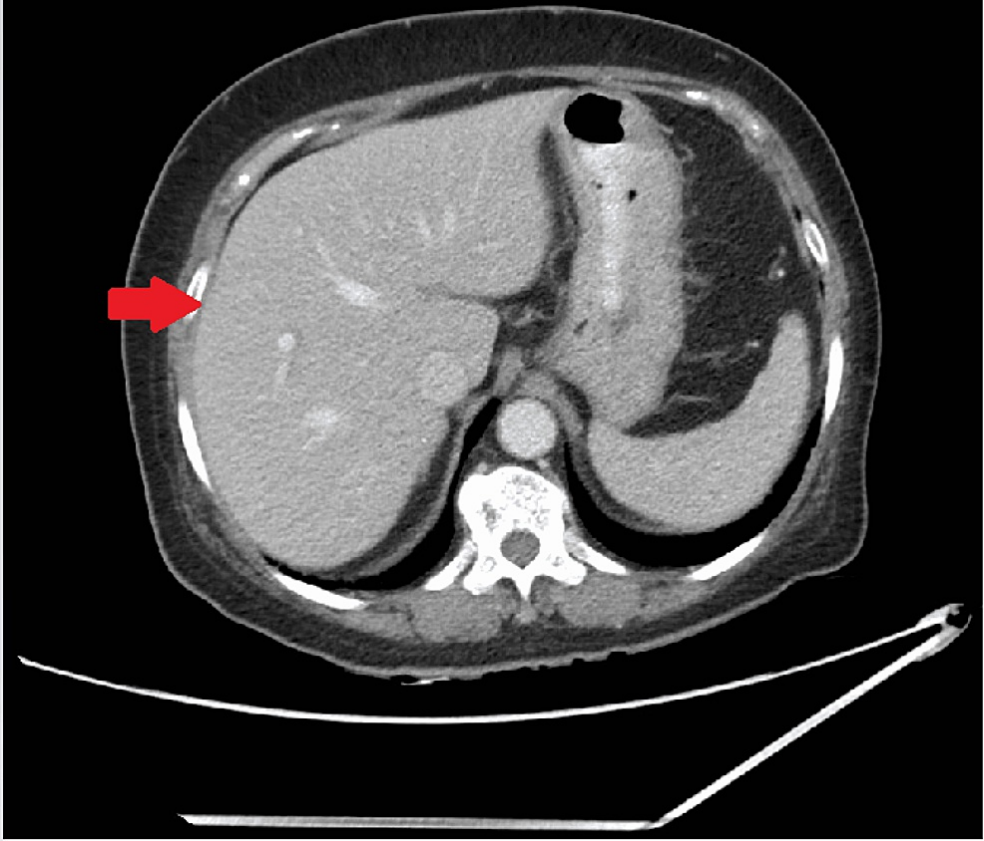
Unremarkable liver visualized on computed tomography of the abdomen and pelvis with no hepatic nodules/lesions noted.
